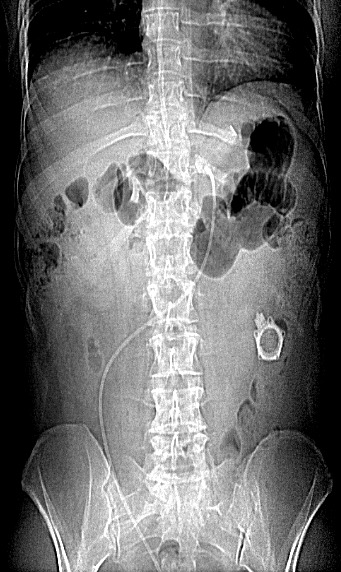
Abdominal X-ray showing disconnected tube from the port, with tube slipping in the pelvis
